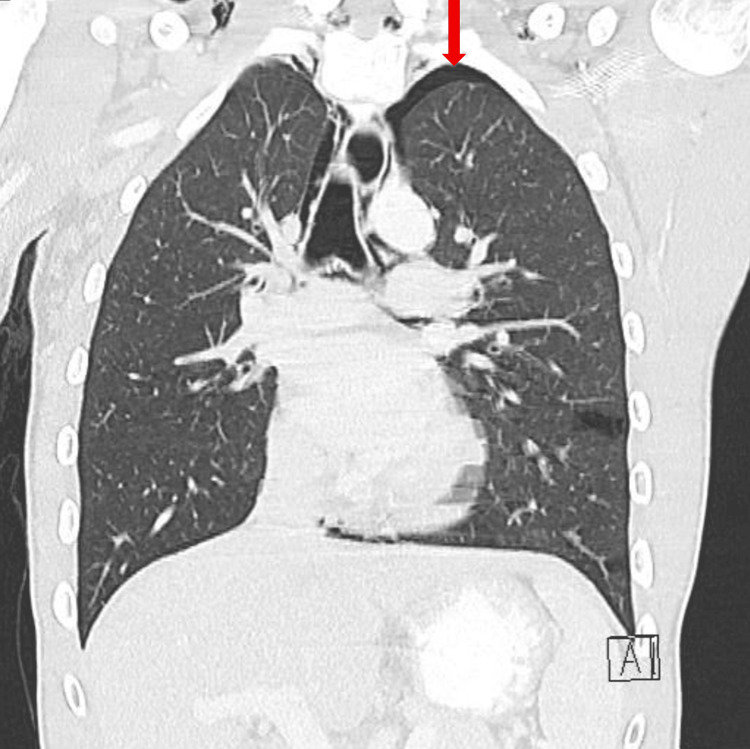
Computed Tomography (CT) scan of the chest (coronal view) demonstrating small left apical pneumothorax (less than 20%) (red arrow).
